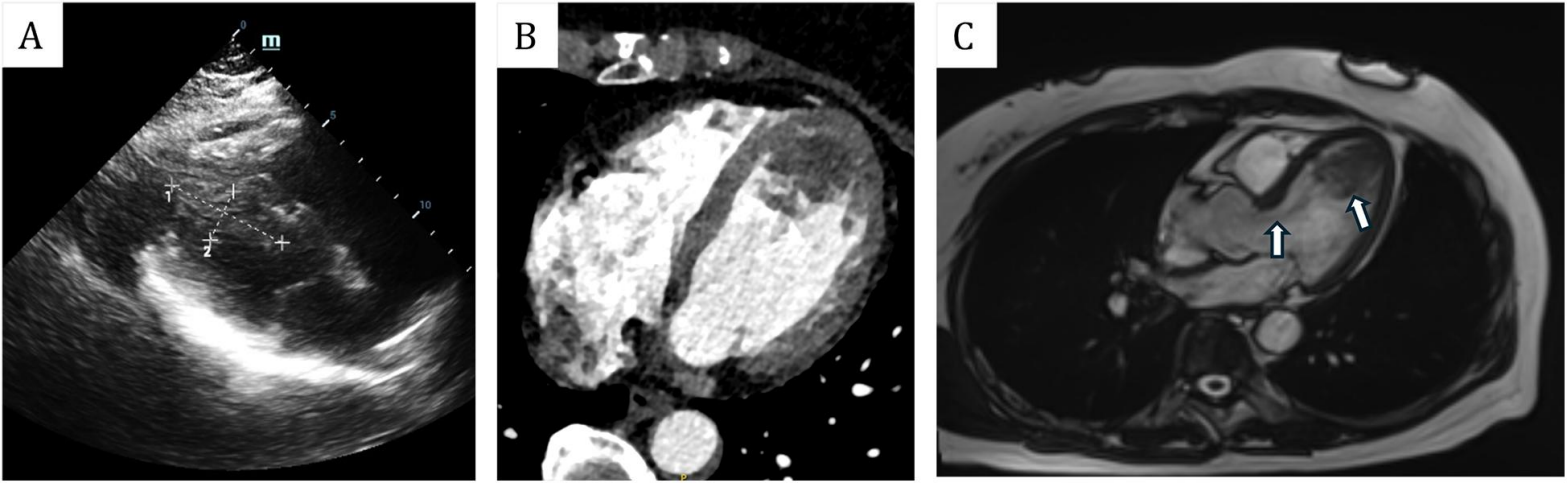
(A) Transthoracic echocardiogram showed that the mass was located in the left ventricle, measuring approximately 49 mm × 21 mm. (B) Enhanced cardiac computed tomography (CT) revealed an irregularly shaped patchy filling defect within the left ventricle. (C) Magnetic resonance imaging (MRI) revealed that the mass was situated in the left ventricle, with its pedicle attached to the ventricular septum. Additionally, flocculent materials were observed extending from the main body of the mass to the aortic valve orifice.
